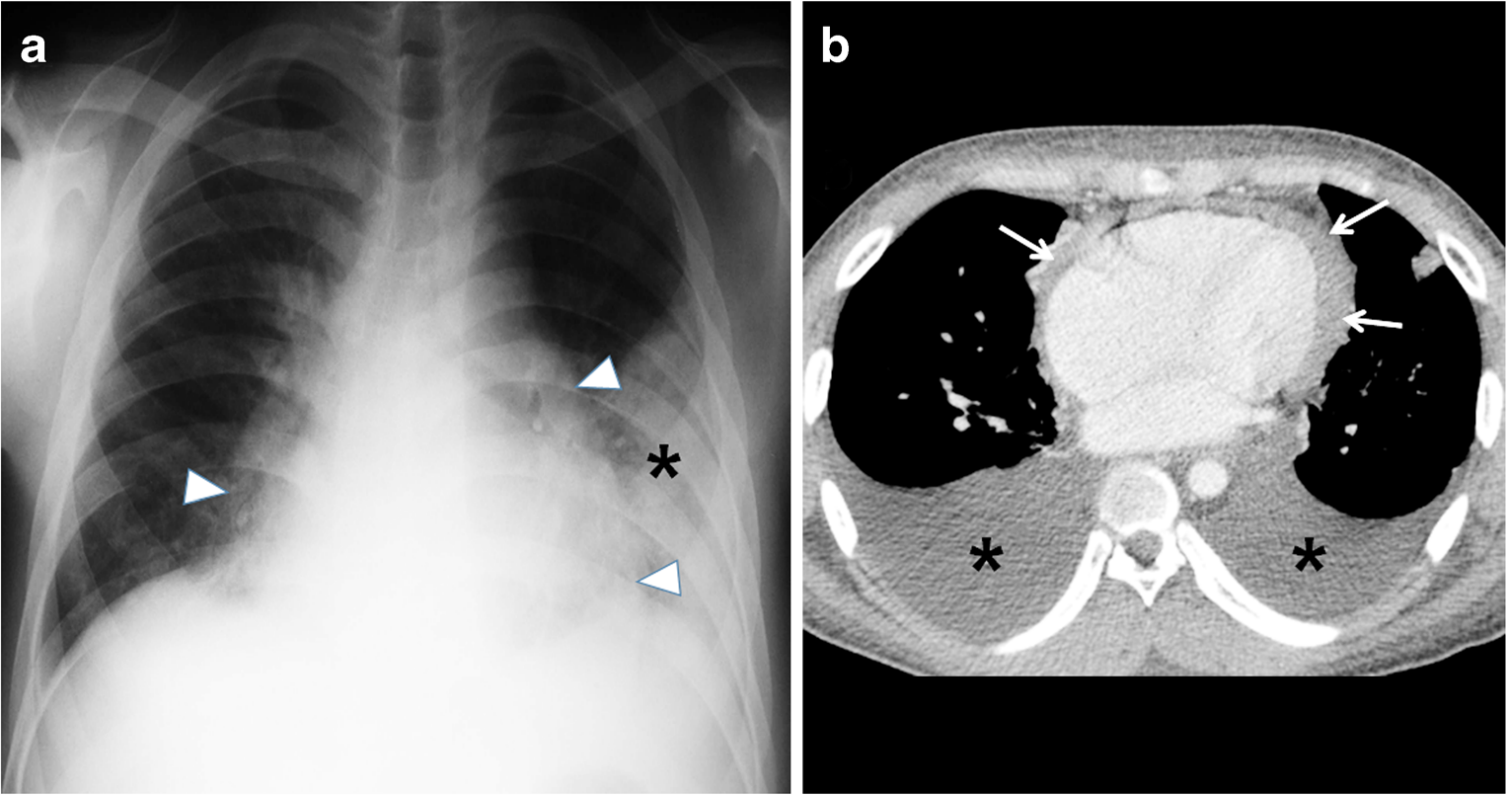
Pleural effusion and cardiac involvement in a 10-year-old girl with primary progressive tuberculous disease. a Posteroanterior chest radiograph shows an enlarged cardiac shadow (*arrowheads*) with pleural effusion (*asterisk*) on the left. b Follow-up axial contrast-enhanced CT image demonstrates bilateral pleural effusions (*asterisks*) and pericardial effusion (*arrows*)
